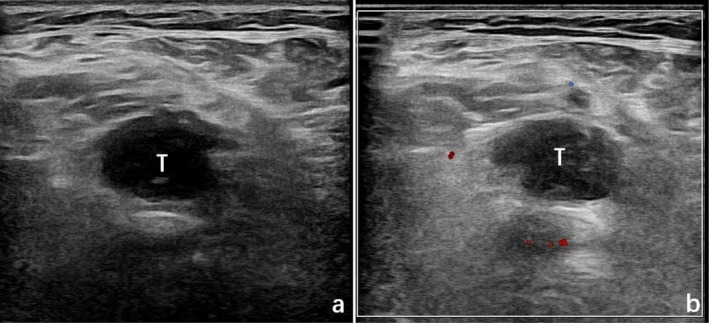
Extradigital glomus tumor in a 59‐year‐old male (left popliteal fossa). (a) Transverse grayscale ultrasound image shows an irregular hypoechoic lesion (T) within muscular tissue exhibiting posterior enhancement. (b) Absent vascularity on color Doppler.
